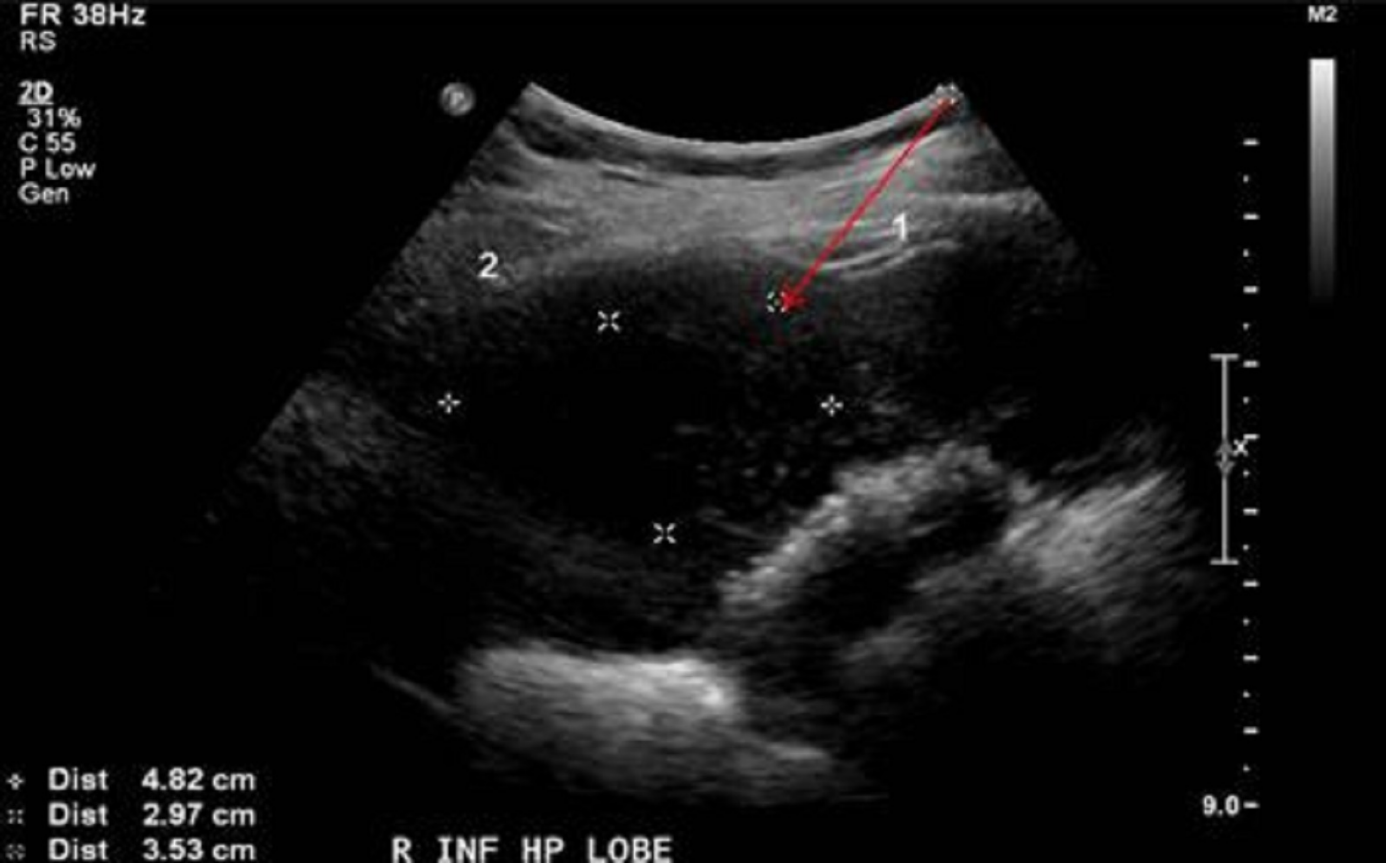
Preparatory imaging of ultrasound guided biopsy in the companion case. The image demonstrates the target lesion and the planned route of the needle (red arrow). The biopsy results revealed a primary biliary cancer, most likely gallbladder carcinoma.
